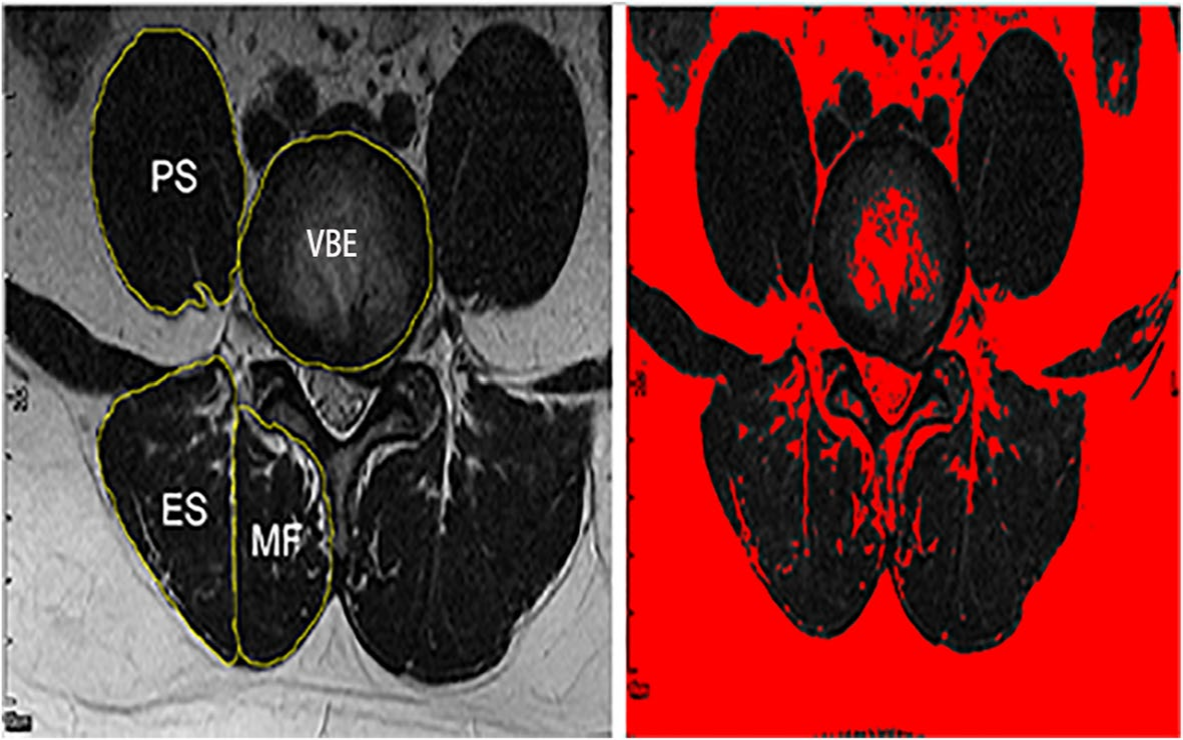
Axial T2-weighted MRI was used to measure the total muscle CSA and fat infiltration rate of the paraspinal muscles at the intervertebral disc level bilaterally. MF: Multifidus ES: Erector Spinae PS: Psoas VBE: vertebral body endplate. The red region of the muscular compartment represents fat
